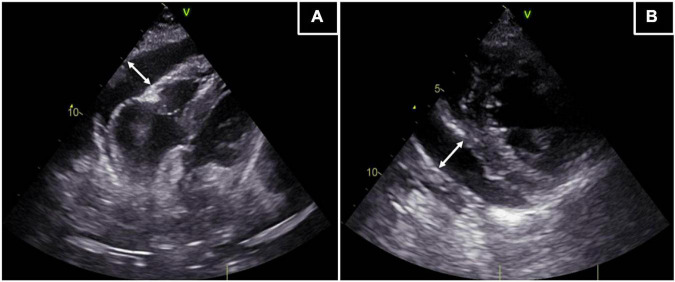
Transthoracic echocardiography. The TTE reveals a circumferential PE of moderate entity (max 17 mm). (A) Off-axis 4 chamber apical view of the epicardial effusion. (B) Parasternal short axis view of the epicardial effusion. White lines with arrows: extension of the PE.
